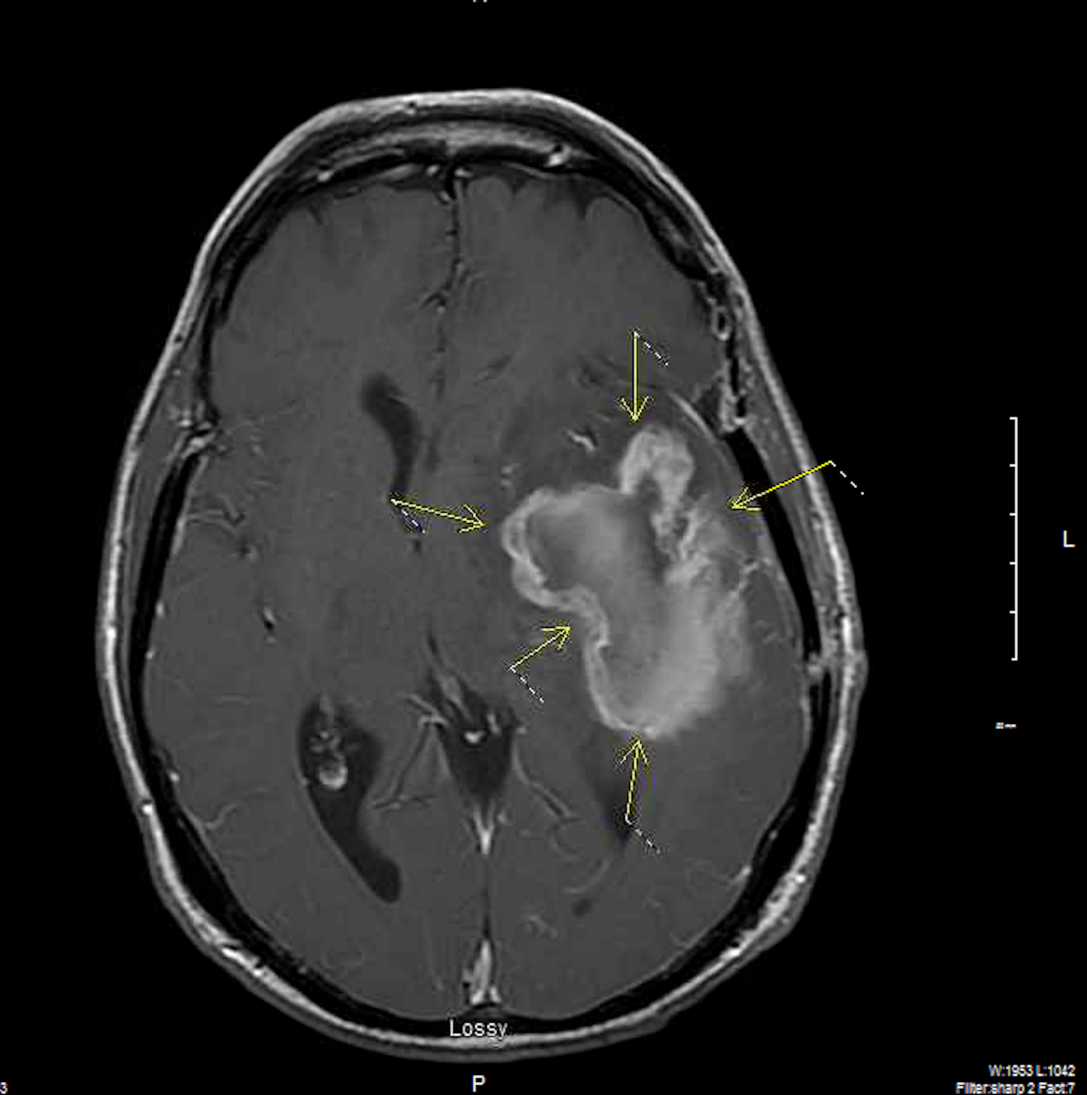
Brain MRI, Axial View with Contrast Enhancement Peripheral enhancement of the left temporal lobe operative bed and associated vasogenic edema consistent with residual or recurrent neoplastic change.
